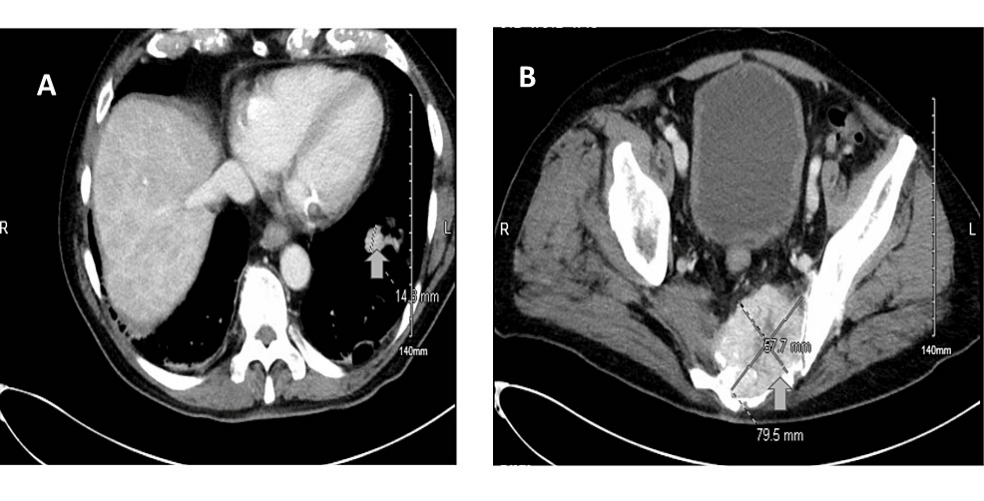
CT abdomen and pelvis with and without contrast revealed (A) pulmonary nodule and (B) 7.9 cm enhancing destructive pelvic mass involving the bilateral sacrum extending into the left pelvic sidewall.
